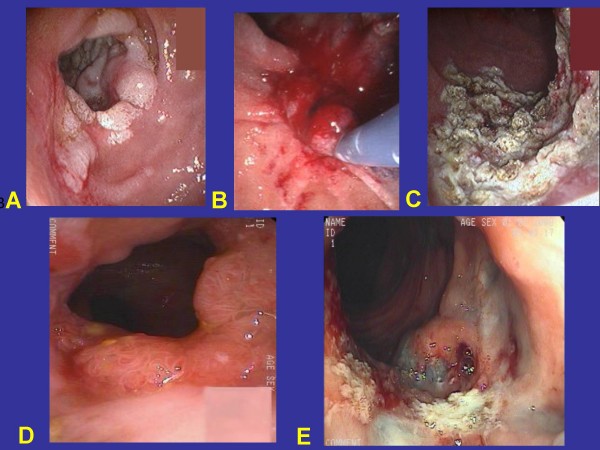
Panel A shows flat and elevated duodenal adenomas in a FAP patient. These were proven by biopsy and endoscopic ultrasound to be free of cancer. Panel B - the polyps were removed by submucosal resection and polypectomy. Panel C - the polyp remnants had been destroyed by argon plasma coagulation (figures provided by Dr. M. Santo, Tel Aviv). Panel D shows a large polyp that developed, during pregnancy, in the ileo-anal pouch of a FAP patient. Panel E - the polyps had been removed by submucosal resection and polypectomy and remnants destroyed by argon plasma coagulation (figures provided by Dr. Z. Halpern, Tel Aviv).
